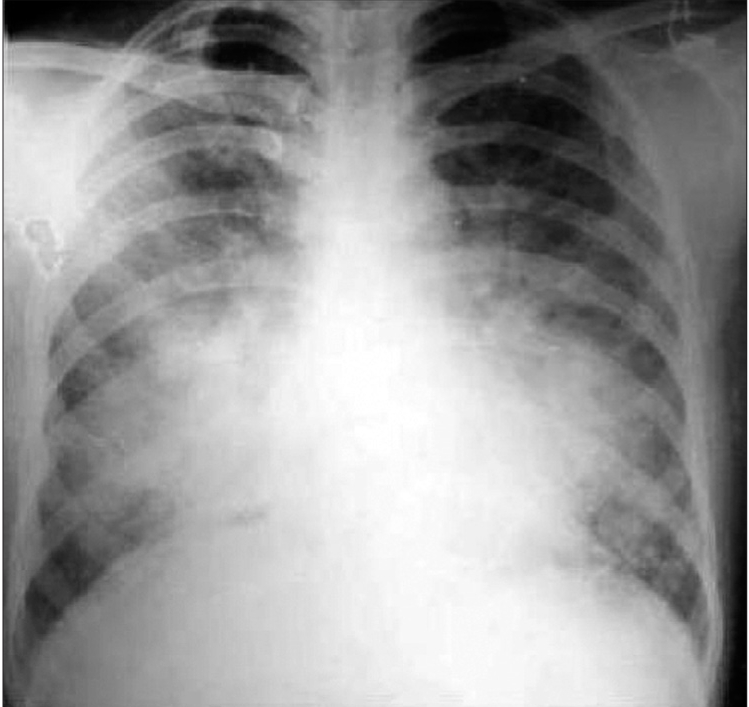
Chest X-ray PA view of Case 1, showing bilateral para-Hilar alveolar filling pattern without cardiomegaly
